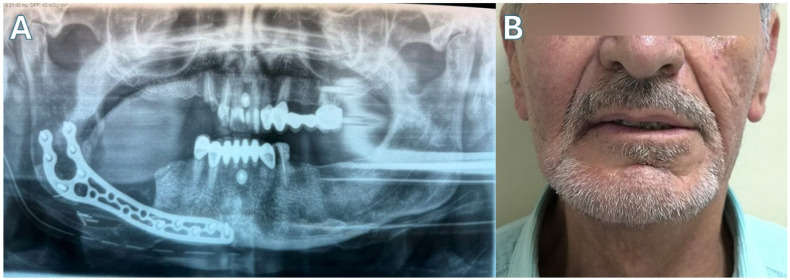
Case 4. (A) Post-op X-ray. (B) The patient 3 months after surgery.
